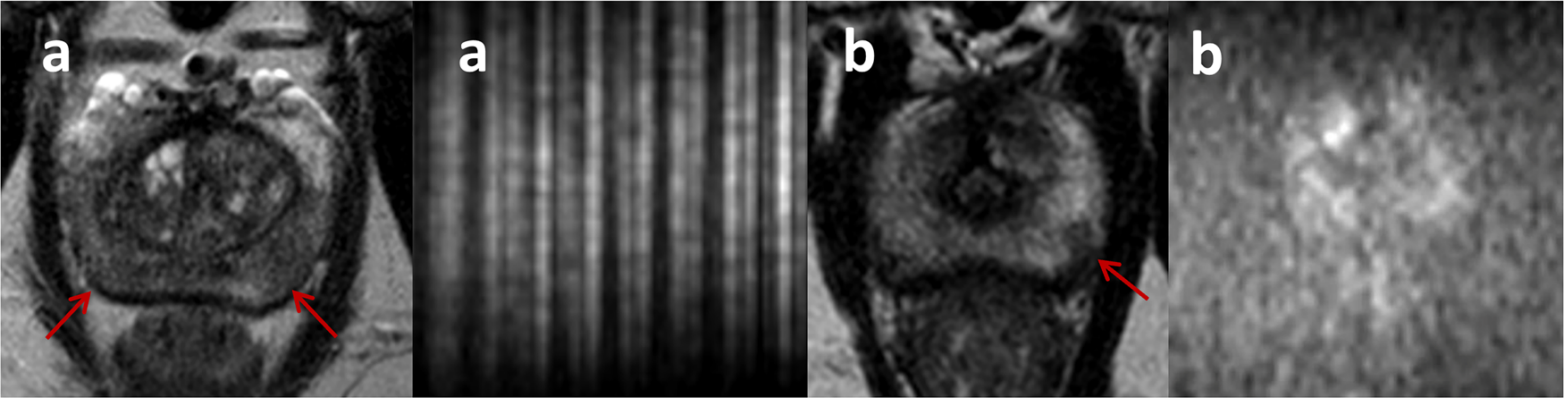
Examples of screening MRI-false-negative scans - paired T2WI (left) and b2000 DWI (right) for each participant (a, b): a 62-year-old male with PSA 6.42 ng/mL and PSAd 0.27 ng/mL^2^. Screening MRI was negative by ReIMAGINE criteria due to diffuse 3/5 change in the peripheral zones (PZ) and non-diagnostic DWI (due to coil malfunction). Biopsy showed right PZ Gleason 4 + 3 and left PZ Gleason 3 + 4 tumour (arrows); b 57-year-old male with PSA 9.22 ng/mL and PSAd 0.26 ng/mL^2^. The screening MRI was reported as negative. Retrospectively classified as a borderline undercall due to focal low signal on T2WI and equivocal changes on DWI in the left PZ at 4/5 o’clock, which was Gleason 3 + 4 on biopsy (arrow)
